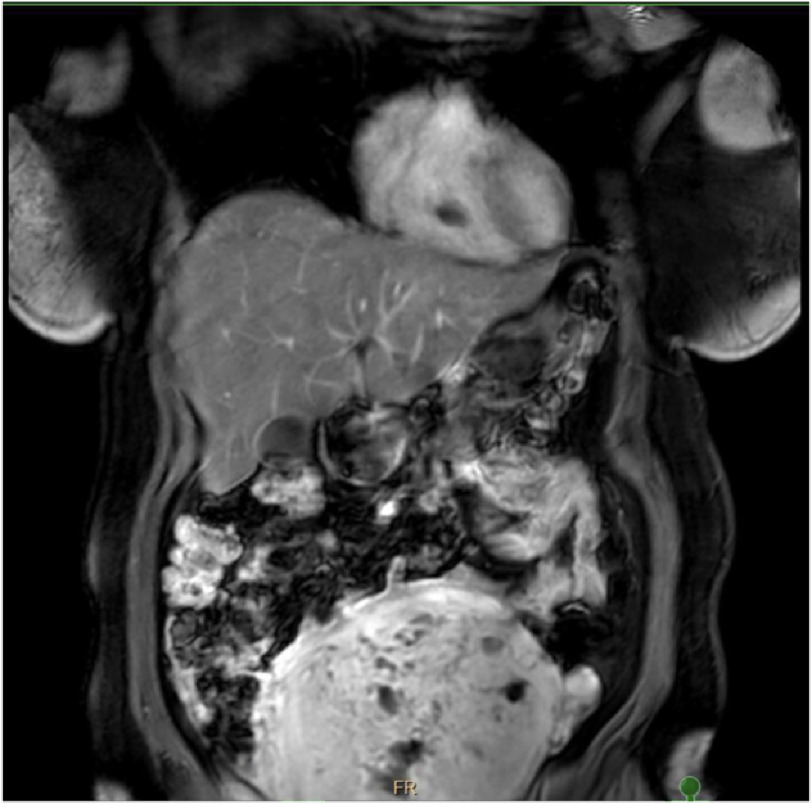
MRI AP with contrast shows an enlarged uterus with numerous cystic spaces consistent with diffuse uterine adenomyosis. Non-enhancing intra-cardiac mass in the right ventricle.
